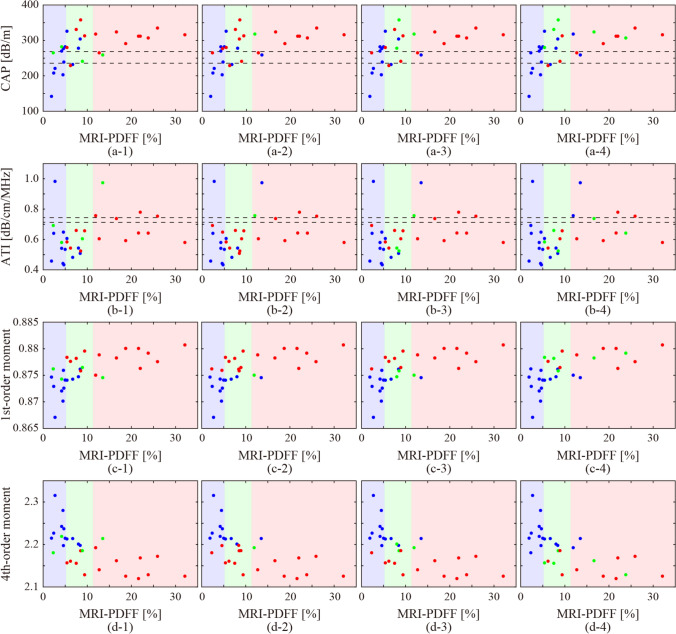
Relationship between the CNN classification of (1) colorized ultrasound images, (2) first-order moment maps, (3) fourth-order moment maps, and (4) moment heatmaps, with other indicators for liver steatosis, a CAP, b ATI, and the means of c first- and d fourth-order moments
